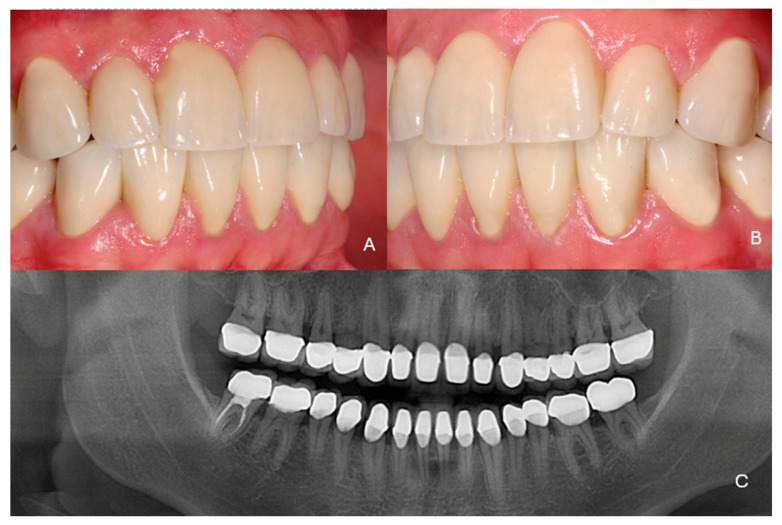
Clinical and radiographic examination at the 5-year recall. (A,B) Side views, (C) radiographic examination.
